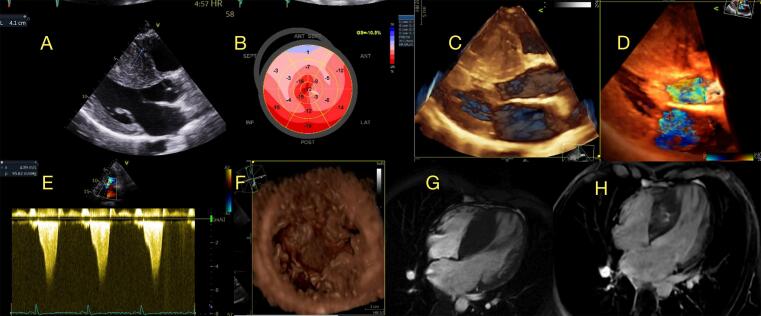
Exemplo de paciente com Cardiomiopatia Hipertrófica Obstrutiva. A) espessura do septo: 4,1 cm, B) Padrão de deformação longitudinal “bulls-eye” mostrando alteração regional principalmente nos segmentos septais e, C) Aquisição de eco transtorácico (ETT) 3D, imagens 3D renderizadas longitudinais mostrando movimento sistólico anterior (SAM) da valva mitral na sístole (*), D) Aquisição de ETT 3D, imagens 3D coloridas renderizadas (visão longitudinal) mostrando regurgitação mitral secundária a SAM (*), E) Doppler contínuo mostrando gradiente de pico tardio em repouso na via de saída do ventrículo esquerdo de 96 mmHg, padrão clássico em forma de adaga, F) Aquisição de ETT 3D, imagens 3D renderizadas (visão de eixo curto) mostrando anomalia dos músculos papilares com quatro cabeças (*) e posicionados anteriormente, G) RMC mostrando hipertrofia septal maciça, e H) imagens com realce tardio com gadolínio mostrando fibrose septal com padrão de parede média.
